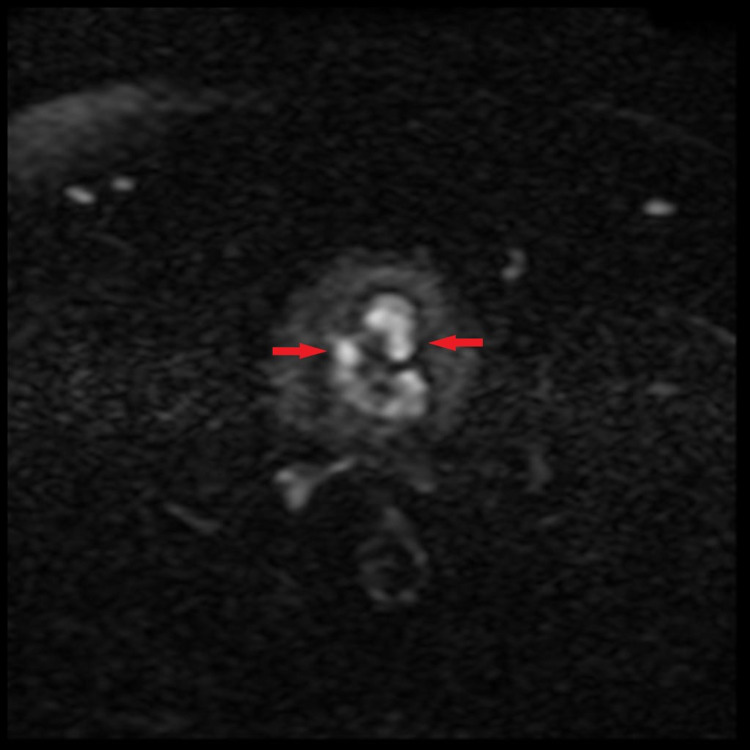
DWI sequence showing diffusion restriction (high signals) within prostate gland (arrows) consistent with prostatic carcinoma. DWI: diffusion-weighted imaging.
